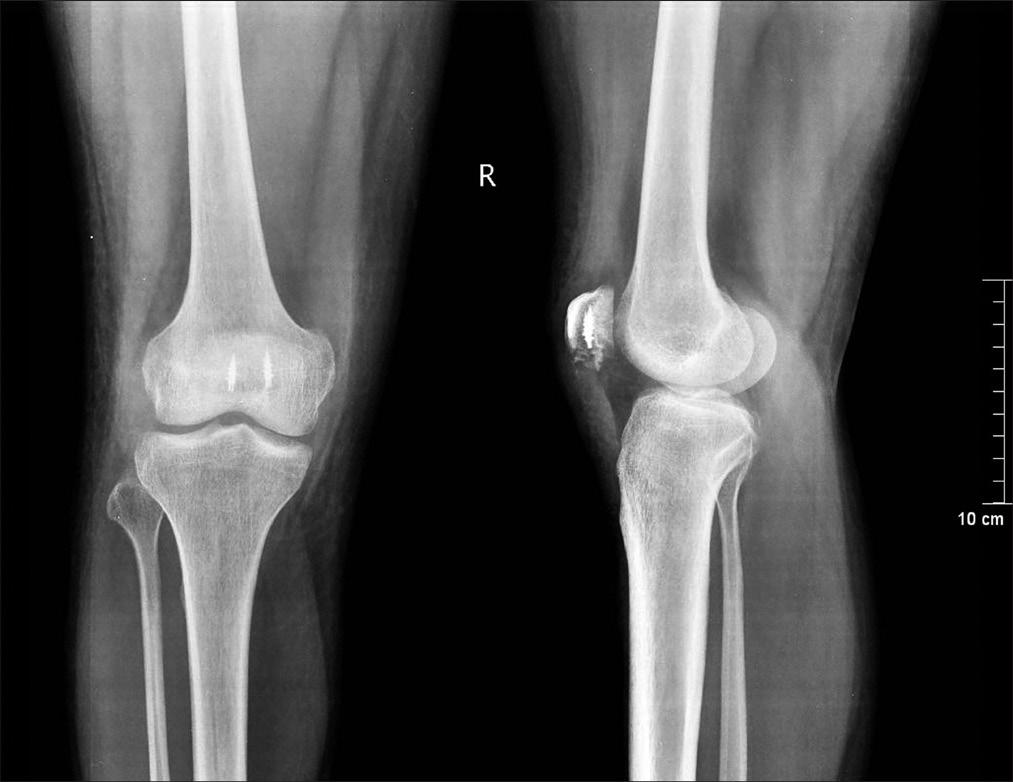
X-rays (anteroposterior and lateral) at final follow- up showing anchors in place and maintained patellar height
